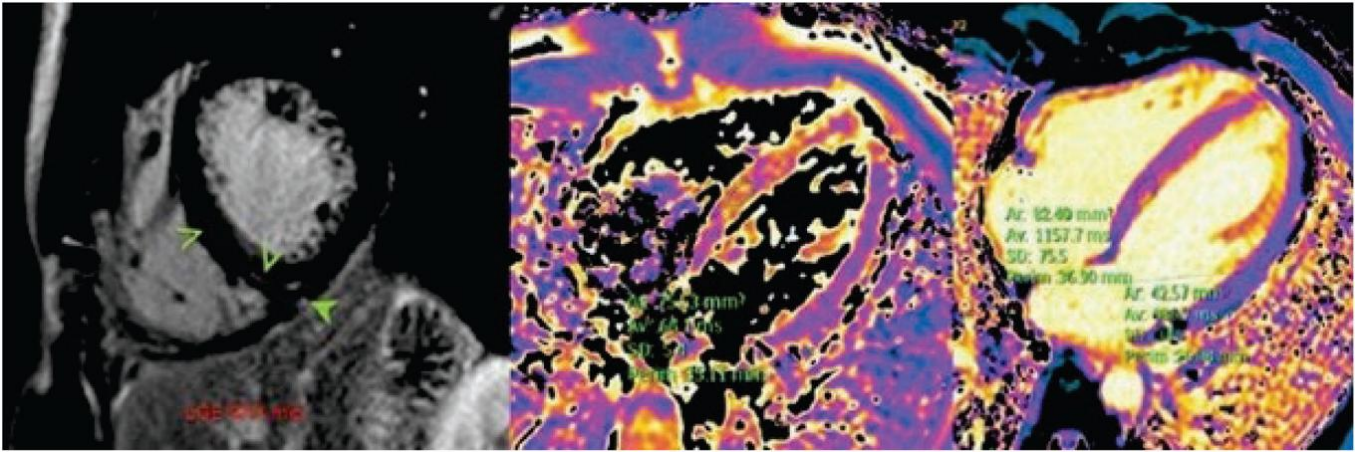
Representative images of the cardiac MRI of the patient. Delayed gadolinium enhancement sequences shown in the figure.
